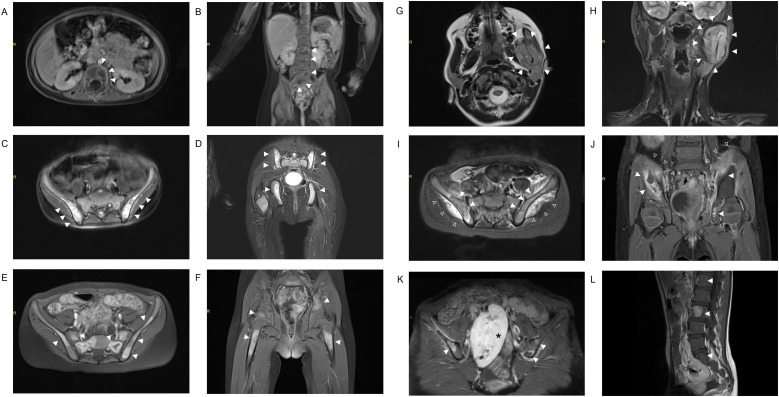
MRI findings in the reported acute leukemia cases (#01–#06). Case #01: axial (A) and coronal (B) plane of a T1-weighted imaging (WI) showing subtle, focal intraosseous contrast enhancement (◄) in the vertebral bodies L2 (A, B), L3 and L5/S1 (B). Slight adjacent soft-tissue changes were observed in the right gluteal region and at the insertion of the left gluteus maximus. The remainder of the skeleton showed physiologic red marrow signal; extremities, joints, and soft tissues were unremarkable. Case #02 (C, D): axial (C) and coronal (D) plane of a T2-WI demonstrating multifocal, sheet-like bone marrow edema (◄) involving the medial ilium bilaterally and the pubic bone. Mild adjacent soft-tissue involvement was suspected, including subtle edema in the gluteus maximus muscles. No significant joint effusions were present in the hips, knees, or ankle joints. Case #03 (E, F): axial (E) and coronal (F) plane of a contrast-enhanced T1-WI showing patchy bilateral enhancement (◄) of the medullary cavity of the ilium, the lateral sacrum, and the proximal femoral diaphysis without involvement of the metaphysis or epiphysis. Case #04 (G, H): axial (G) and coronal (H) plane of a T2-WI showing an osteodestructive, highly cellular, heterogeneously enhancing mass of the left mandible (◄) extending from the condylar head and coronoid process to the mandibular ramus. The lesion infiltrates large portions of the left masticatory musculature. Case #05 (I, J): axial plane (I) of a T2-WI and coronal plane (J) of a T1-WI with contrast showing multifocal marrow abnormalities with peripheral edema signal and peripheral contrast enhancement of the medial ilium with central hypointense signal of the medullary cavity (◄) and edema of the adjacent gluteus medius (empty◄) and iliacus muscle. Case #06 (K, L): pelvis-oriented plane (K) of a post-contrast fat-suppression (FS) T1-WI and sagittal plane of a post-contrast FS T2-WI (L) displaying multiple areas of abnormal signal (◄) involving the L1, L3, L4, and S1 vertebral bodies and with marked contrast enhancement. The largest lesion was at L3, extending through the entire height of the vertebral body and located predominantly along the right anterolateral aspect. Small areas of abnormal signal with pronounced contrast uptake were also present in both iliac wings (K), with a small hypointense avascular area in the left iliac wing reflecting necrosis. The asterisk (*) indicates known nephroptosis.
